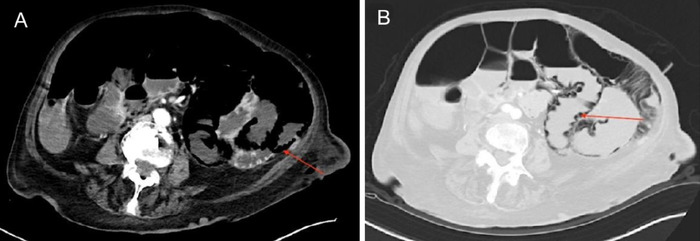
Computed tomography with intravenous contrast (A, B) axial views of the abdomen and pelvis show diffuse pneumatosis intestinalis (arrows) in the small bowel.
